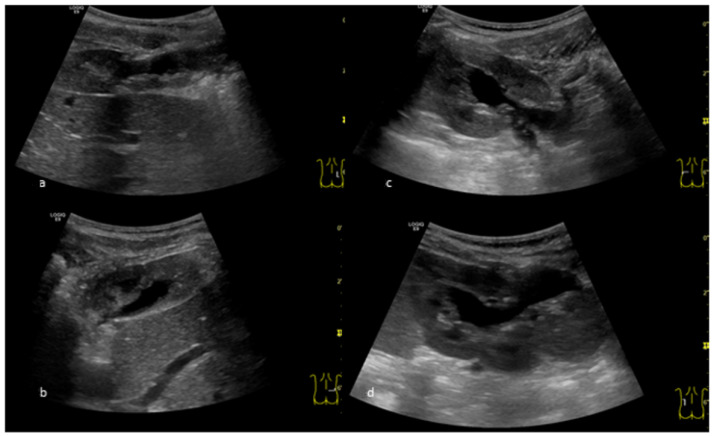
Ultrasound nearly 4 years postoperatively: (a) Right kidney: longitudinal view; (b) Right kidney: cross-sectional view; (c) Left kidney: longitudinal view; (d) Left kidney: cross-sectional view.
